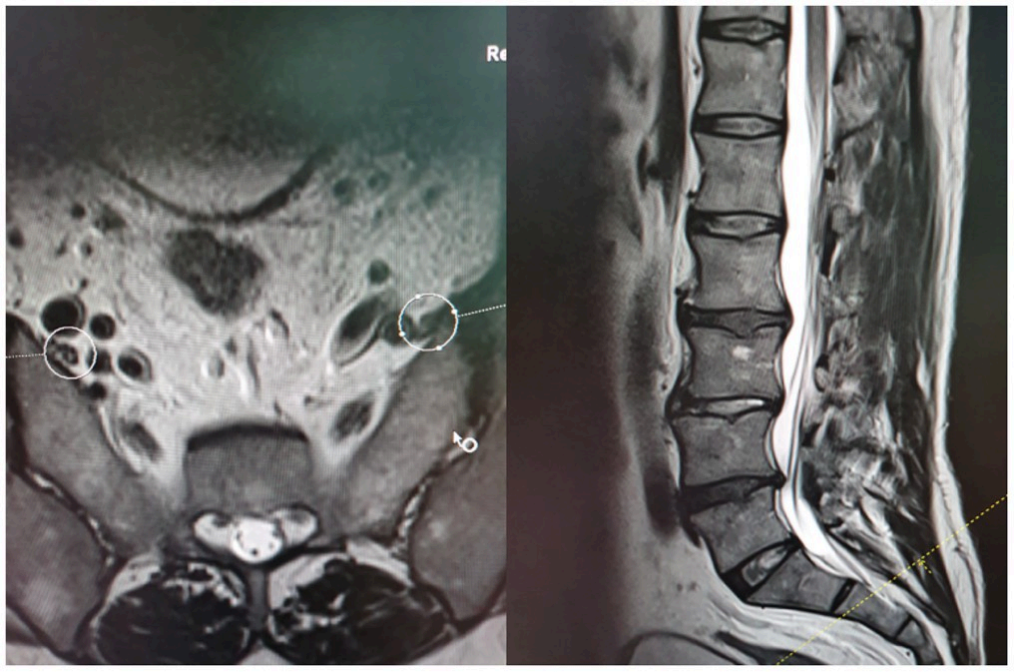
MRI showing transverse and sagittal projections with level of point C of SIJ.
